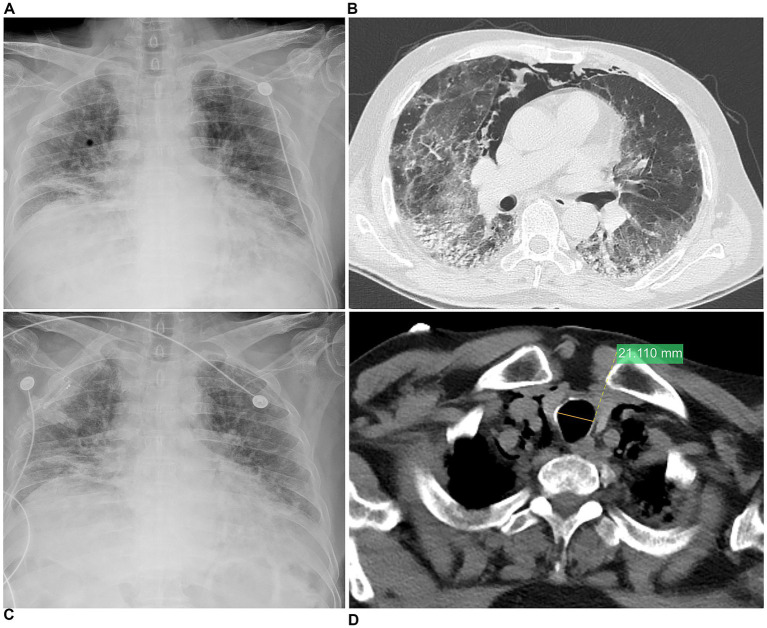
Chest imaging of case 1 (62-year-old male patient, corresponds to typical case 1). (A) Admission chest X-ray showing multiple GGO and patchy infiltrates in both lungs. (B) Day 3, lung window CT showed bilateral GGO, patchy infiltrates, reticular changes, and traction bronchiectasis, most prominent in the lower lobes of both lungs, with SPM. (C) Mediastinal window shows slight tracheal dilation. (D) On the fifth day, bedside chest X-ray showed that multiple areas of GGO and patchy exudation in both lungs, reduction in the extent of right lower lobe linear opacities and left lower field lesions compared to previous imaging.
